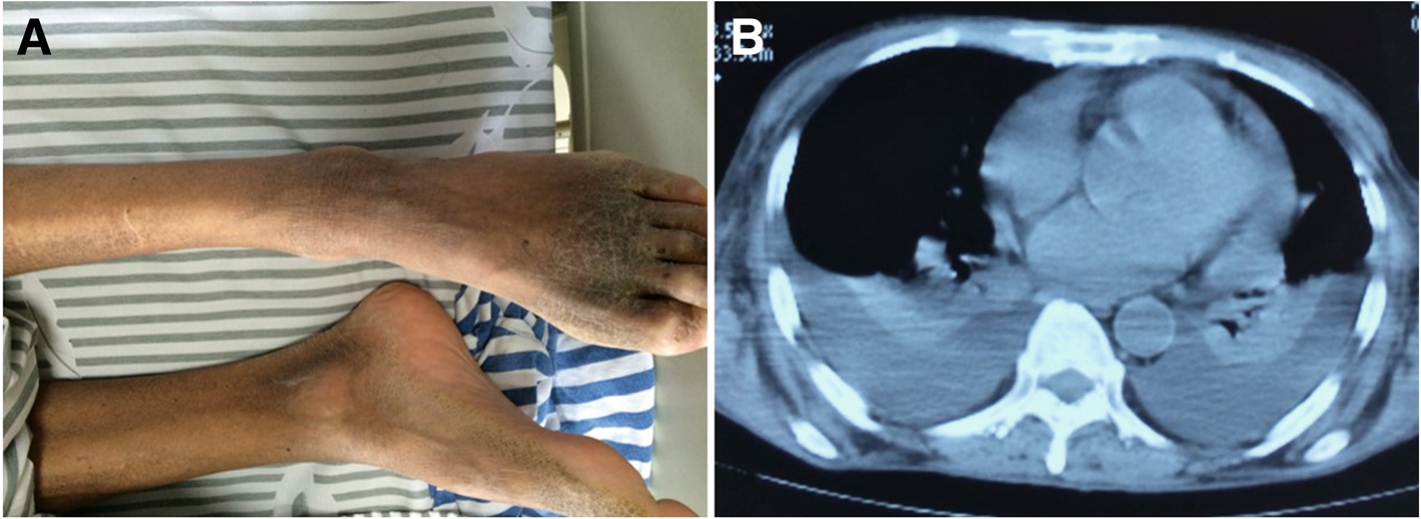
The clinical presentation of POEMS. The pigmentation with skin thickening on feet (a). The thoracic and abdominal CT showing splenomegaly(b)
